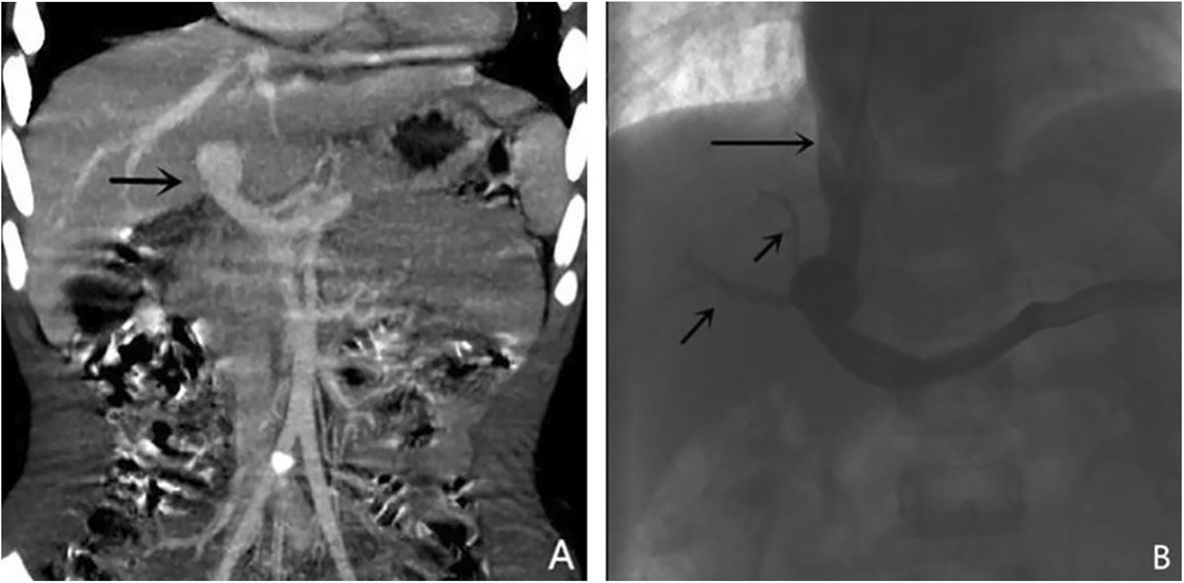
Male, 3 years old, Abernethy II. a: Abdominal CT angiography maximum intensity projection (MIP) image (portal phase) shows the presence of extrahepatic portosystemic shunt (arrow) with absence of intrahepatic portal veins and diagnoses Abernethy Ib;b: DSA image shows the shunt (long arrow) and the tiny intrahepatic portal veins (short arrow)
